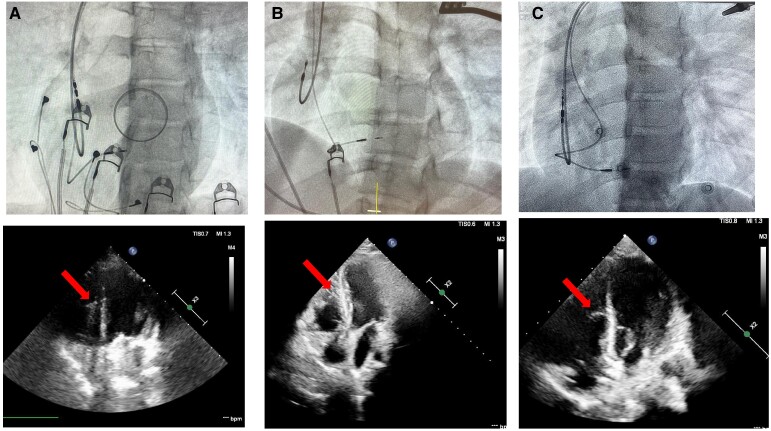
Left anterior oblique view at the end of the procedure and transthoracic echocardiography 1 day after the implantation: Case 1 (*A*), Case 2 (*B*), and Case 3 (*C*). The arrow indicates the right ventricular lead.
